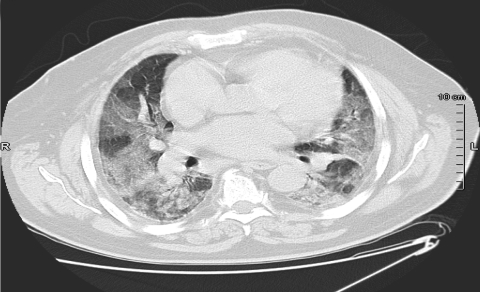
Thoracic computed tomographic scan on day 3, showing bilateral alveolo-interstitial infiltrates.
